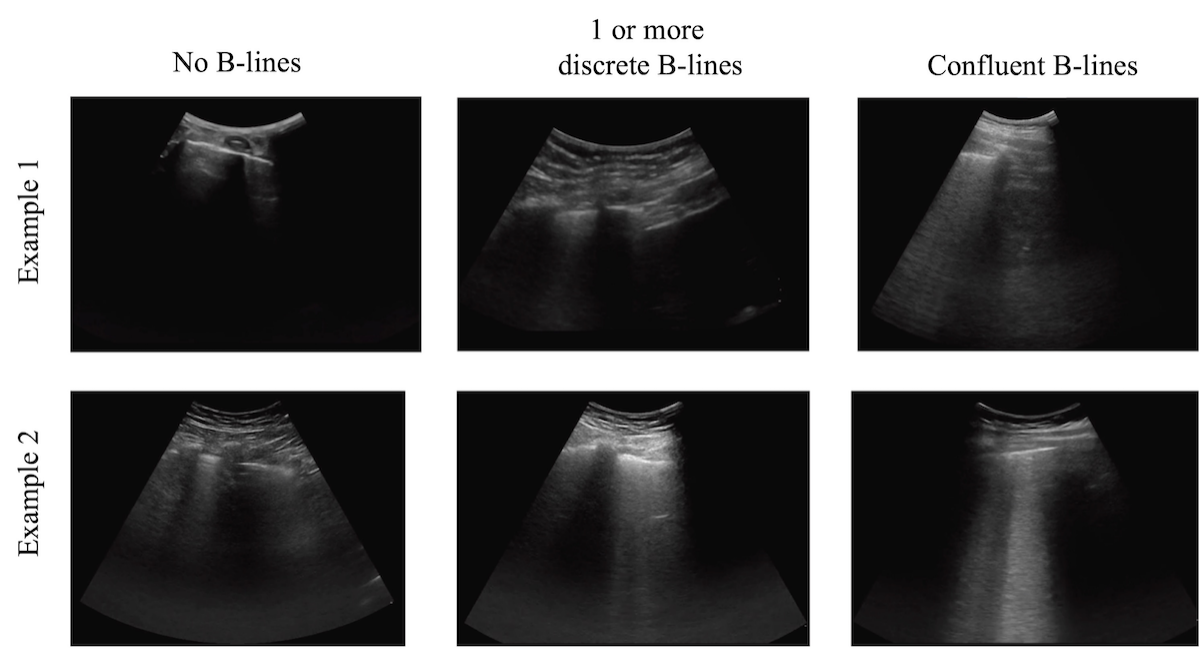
Representative frames from lung point-of-care ultrasound (POCUS) images demonstrating the 3 potential classification label categories. (1) Two lung POCUS images demonstrating no B-lines; (2) Two lung POCUS images demonstrating discrete B-lines; (3) Two lung POCUS images demonstrating confluent B-lines.
